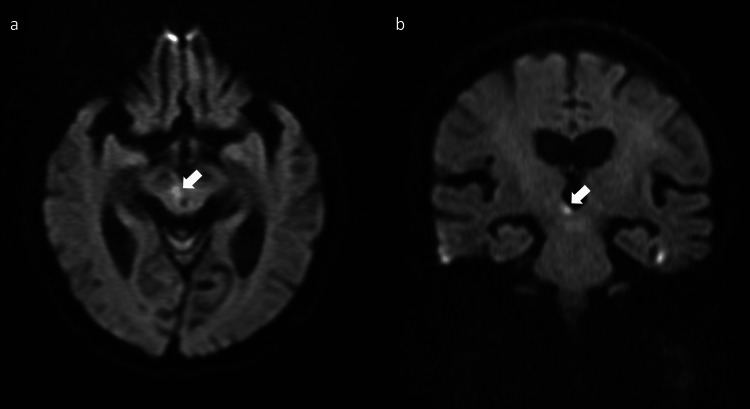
Brain MRI of the present case. Diffusion-weighted imaging demonstrated hyperintensity in the right rostral paramedian midbrain (arrows; a, axial view; b, coronal view).
